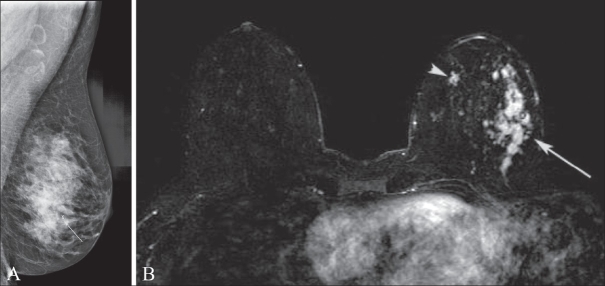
Mediolateral oblique projection mammogram (A) and fat-saturated axial T1W postcontrast (B) images. In this 60-year-old woman who desired breast conservation, DCE-MRI not only demonstrated extensive disease laterally (arrow in B) at the site of the mammographic finding (arrow in A) but also in the medial aspect of the breast (arrowhead in B). The medial breast was biopsied under MRI guidance and there proved to be an additional focus of lobular carcinoma. The surgical management in this case was mastectomy with breast reconstruction
